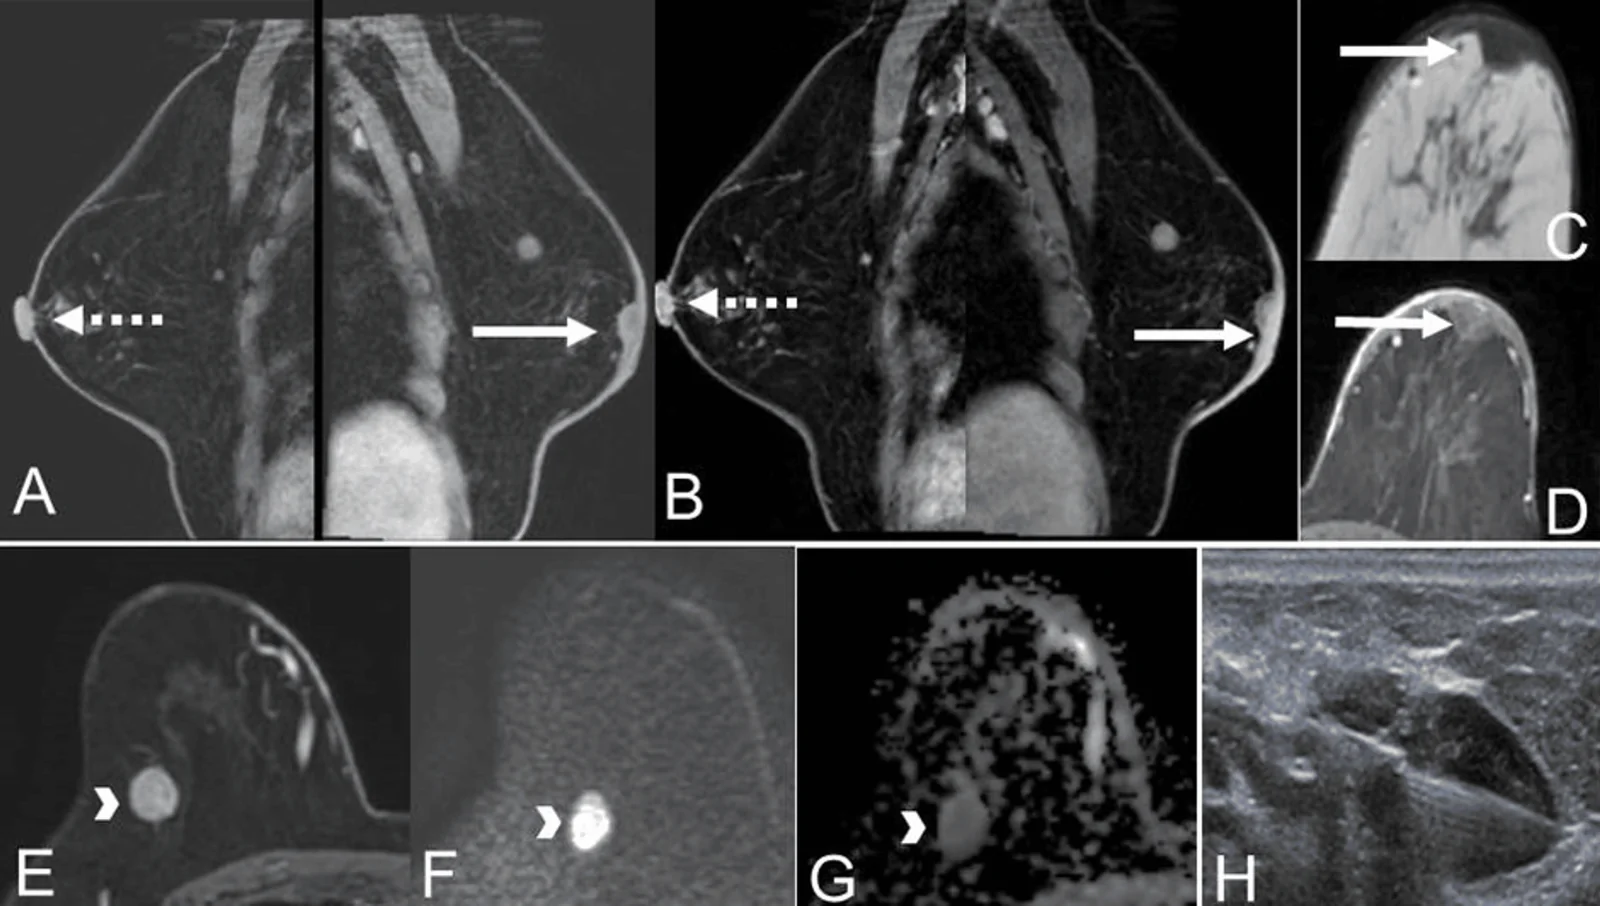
MRI and ultrasound-guided biopsy demonstrating left nipple involvement with an enhancing subareolar mass showing diffusion restriction ADC: apparent diffusion coefficient (A-D) MRI depicting nipple erosion, skin ulceration, subcutaneous edema, focal enhancing subareolar thickening of the left nipple (solid arrow), and the right normal nipple represented by dotted arrows. (E-G) Enhancing circumscribed round mass showing diffusion restriction and ADC signal drop (arrowhead). (H) Ultrasound-guided core needle biopsy from the mass
